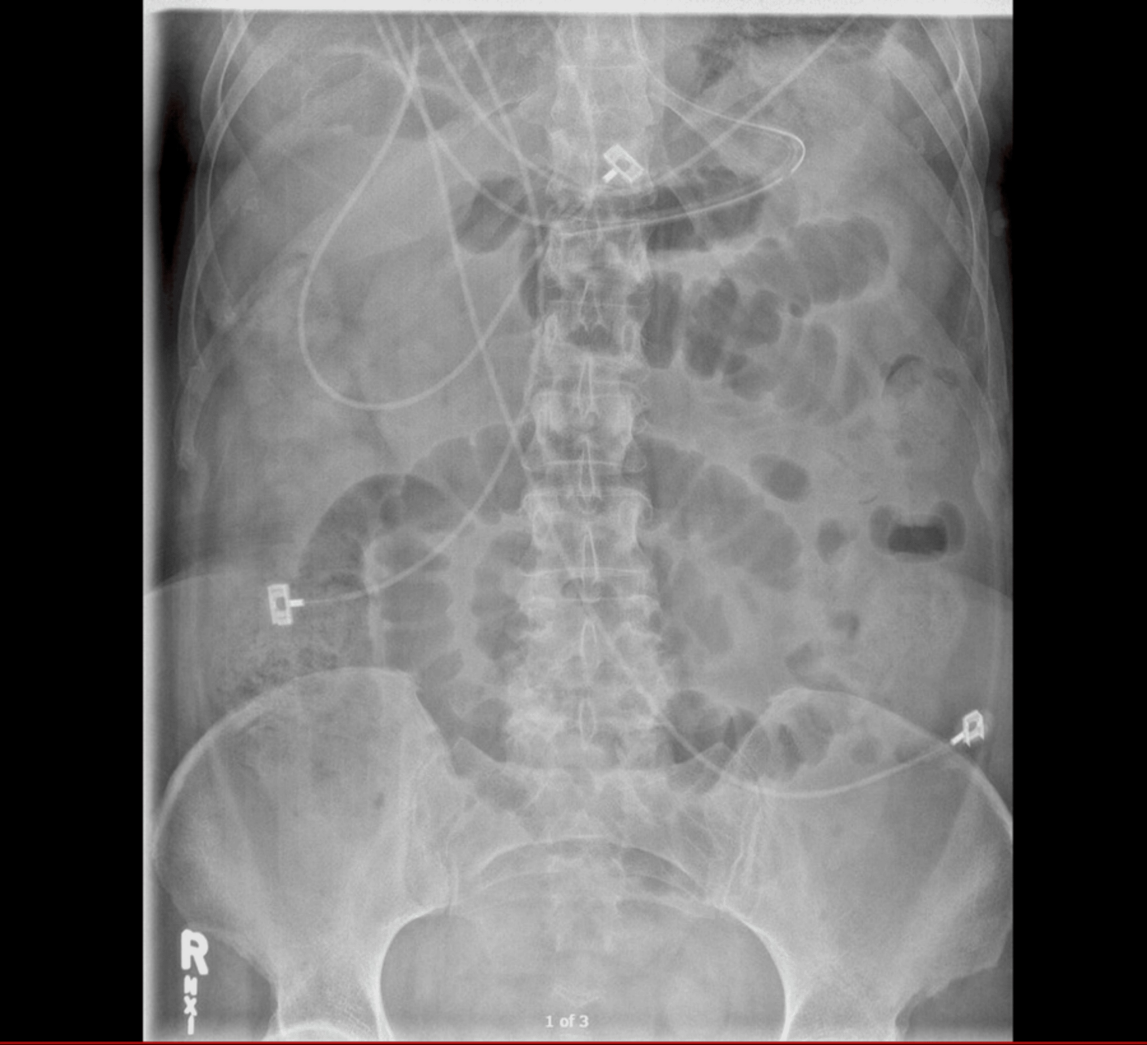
Upright KUB X-ray hospital admission day two KUB: kidney, ureter, and bladder
